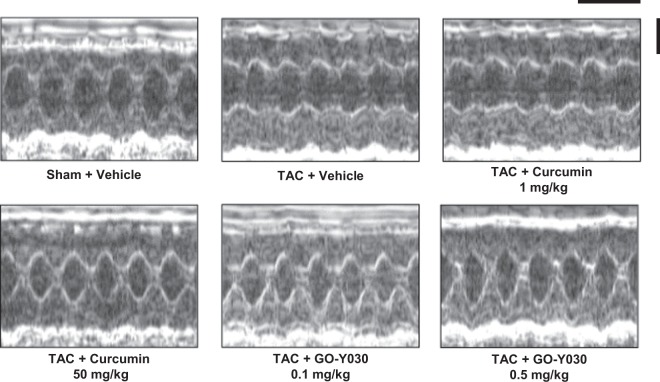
GO-Y030 at a dose 1/100th that of curcumin significantly suppressed TAC-induced cardiac hypertrophy and systolic dysfunction *in vivo*. Six weeks after the operation, cardiac function was assessed by echocardiography. Representative images of echocardiography at 6 weeks after TAC or sham operation. Horizontal bar: 200 ms. Vertical bar: 2 mm.
